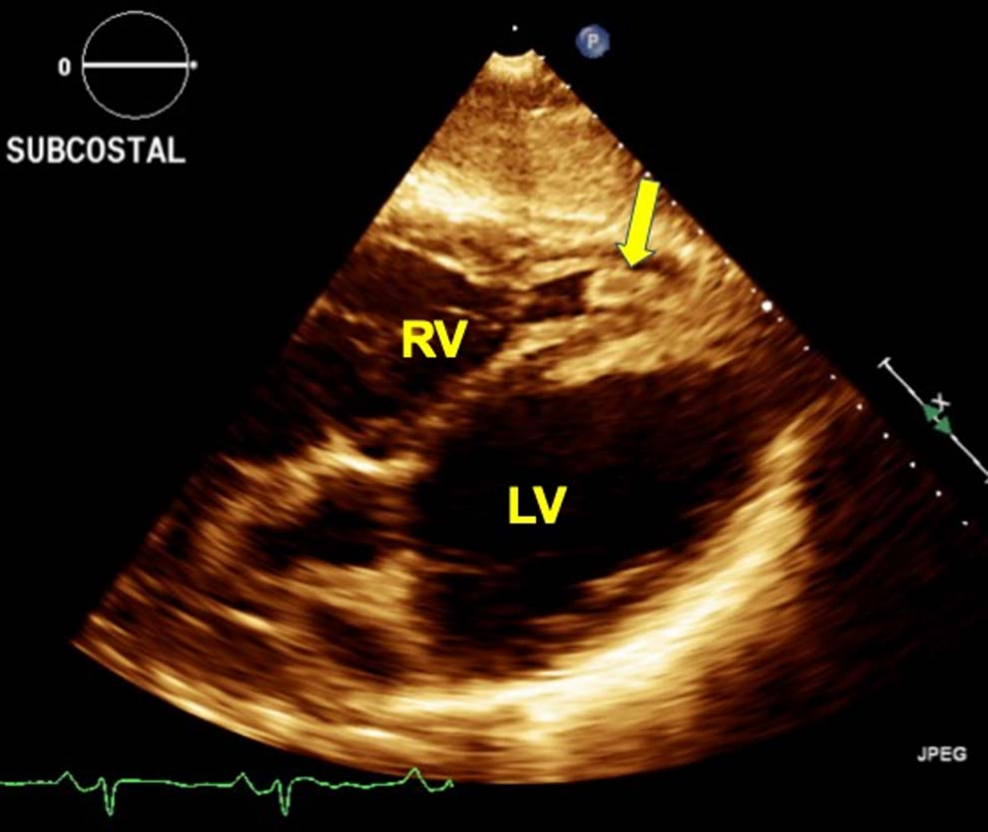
Transthoracic echocardiogram subcostal view demonstrates right ventricle mass close to the apex (arrow). LV, left ventricle; RV, right ventricle.
